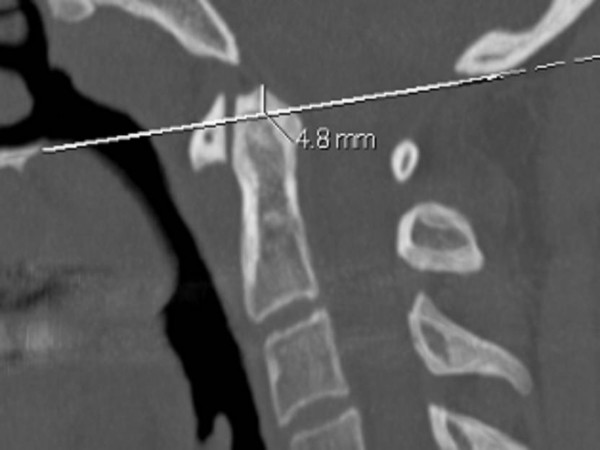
(patient 2) Sagittal reformatted multiplanar computed tomography of the craniocervical junction showed a hyperplastic odontoid process. The tip of the dens projected 4.8 mm above a line joining the back of the hard palate to the lowest point of the occipital squama (McGregor line). A mild basilar invagination was therefore present. Note the increased bone density of the posterior vertebral elements.
